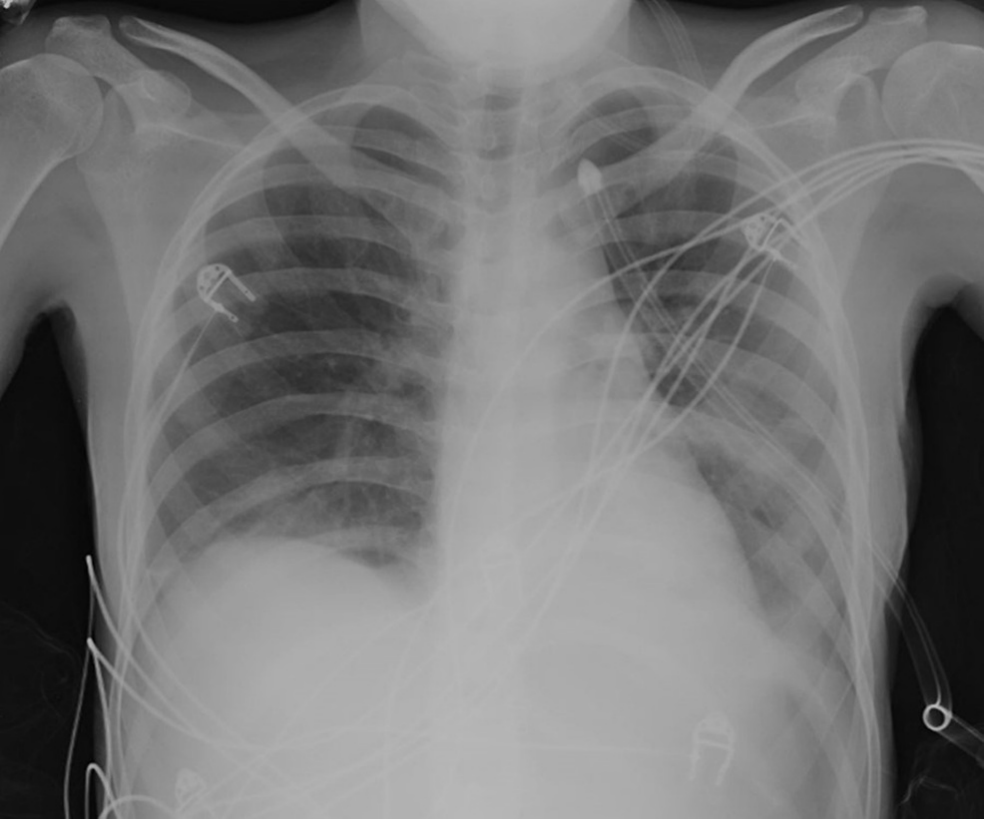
Postoperative chest X-ray Postoperative chest X-ray showing correction of the diaphragmatic hernia.
